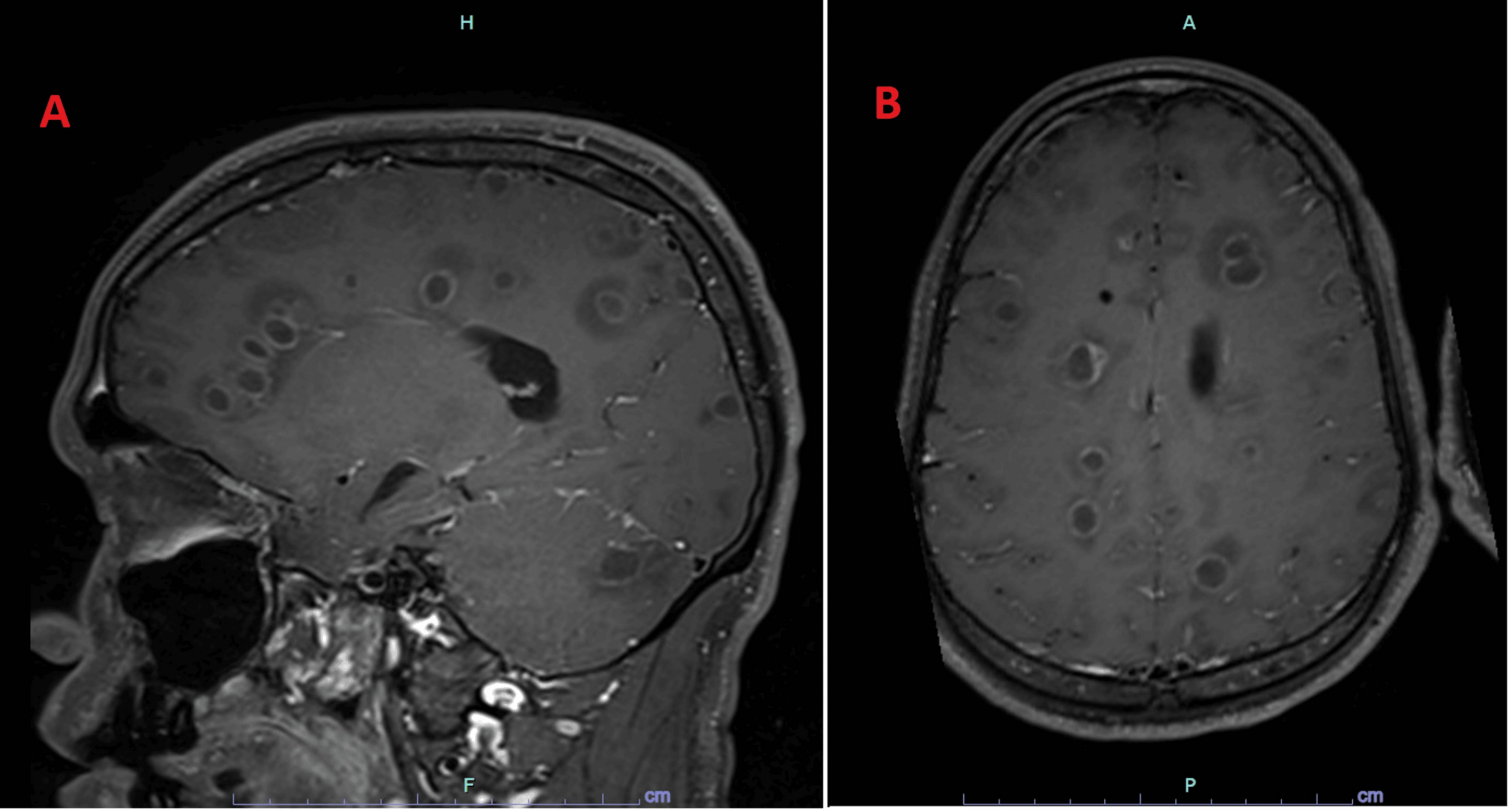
MRI of multifocal bilaterally disseminated lesions. Sagittal (A) and axial (B) MRI demonstrating bilateral lesions disseminated around the lateral ventricles and throughout the supra- and infratentorial regions (T1 3D space G+ MPR). MRI: magnetic resonance imaging; MPR: multiplanar reformation or reconstruction
